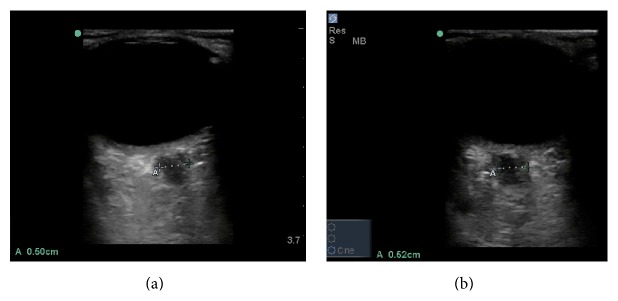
Bedside ocular ultrasound of left (a) and right (b) eyes, showing measurement of optic nerve sheath diameter. Measurements are 5 mm and 5.2 mm, respectively.
